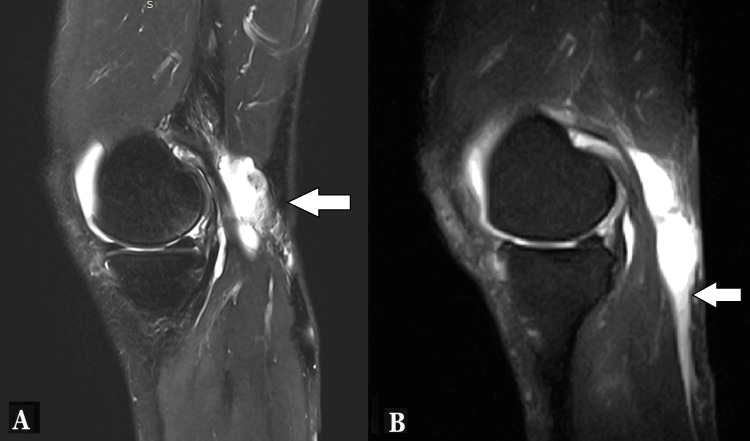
MRI of a ruptured Baker’s cyst. MRI sagittal images of a ruptured Baker’s cyst showing irregular fluid collection (A) with dissection along the fascial plane (B).
